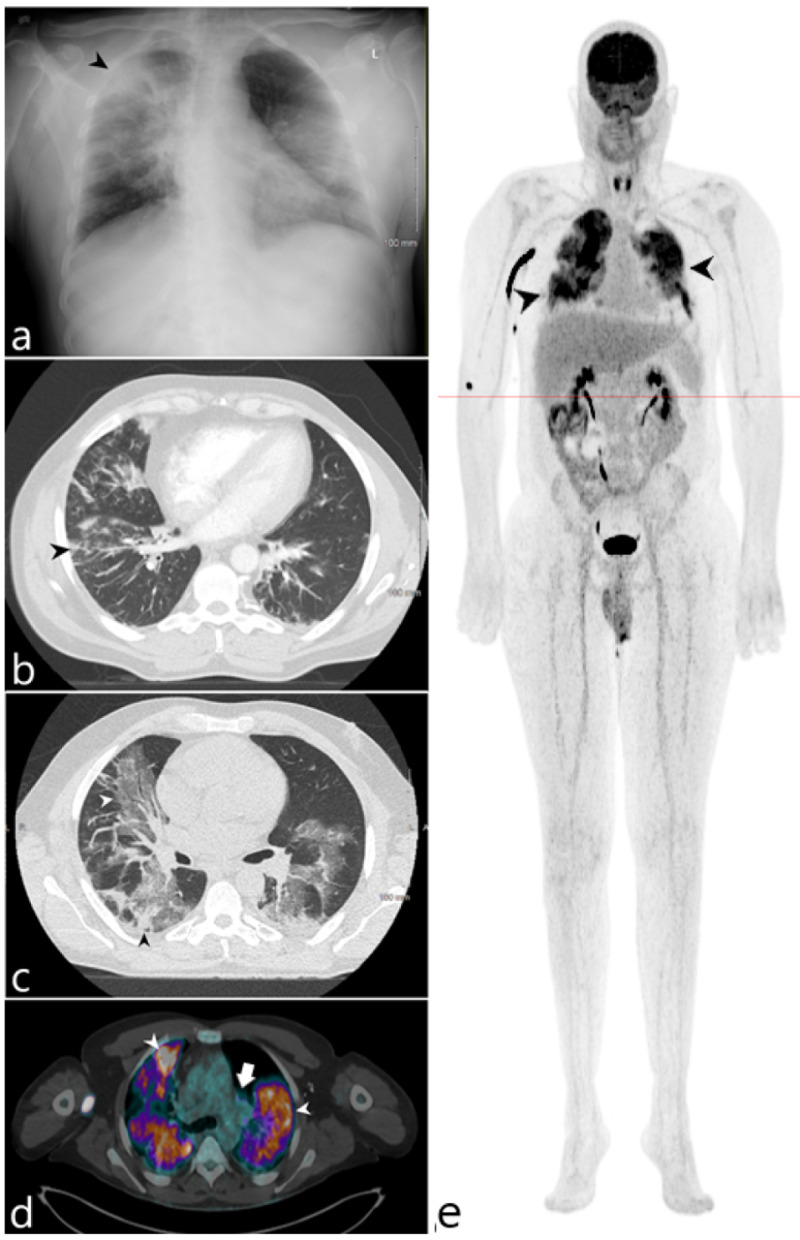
54-year-old male admitted for respiratory failure in the setting of COVID-19 pneumonia (a) Initial admission radiograph demonstrates diffuse opacities with sites of peripheral consolidation (black arrowhead). (b) Axial CT of the lower thorax performed on hospital day 10 demonstrates multifocal peribronchovascular ground glass opacity, prominently within the right lung. (c) Axial CT of the chest acquired on hospital day 22 demonstrates worsening ground glass (white arrowhead) and consolidative opacities (black arrowhead) within both lungs. (d) Fluorine-18 FDG/PET-CT axial fused series demonstrates diffuse intense radiotracer uptake within the lungs (white arrowheads) as well as within the hilar lymph nodes (white arrow). (e) 3D maximum intensity projection image similarly demonstrates intense radiotracer uptake within the lungs (black arrowheads) without additional sites of extrathoracic radiotracer uptake.
